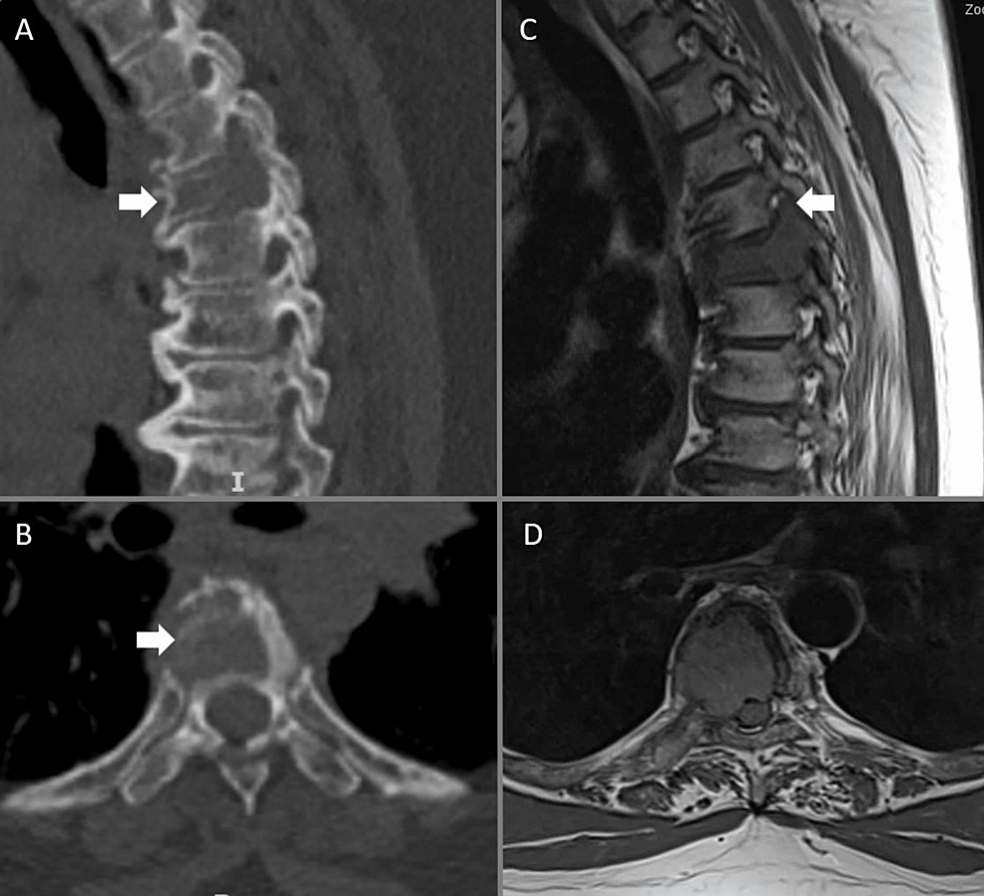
Case two Sixty-three-year-old female with thyroid metastasis. A and B demonstrate a lytic T5 lesion on sagittal and axial CT imaging respectively (arrows). C and D show right parasagittal and axial T1 MRI images. Of note in C tumor can be seen extending along the T5 right pedicle into the superior articular process (arrow).
